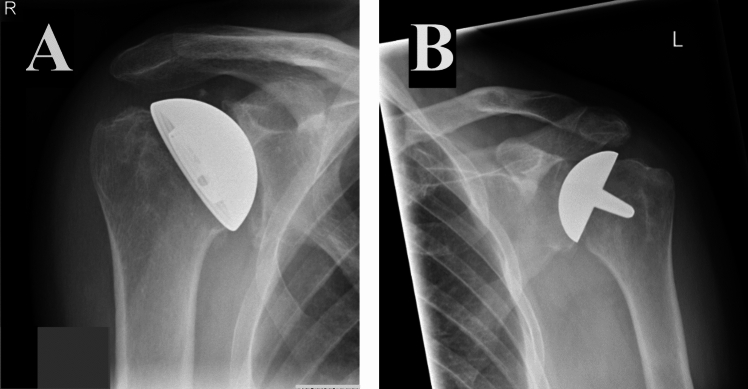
Postoperative radiographs of the Epoca A and Copeland B humeral head resurfacing arthroplasties
